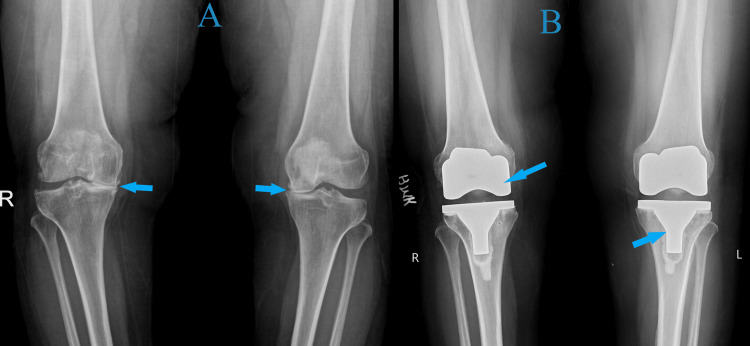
A) Preoperative X-ray showing varus deformity and arthritic bilateral knee joints. B) Immediate postoperative X-ray-aligned and well-positioned total knee arthroplasty implants.
